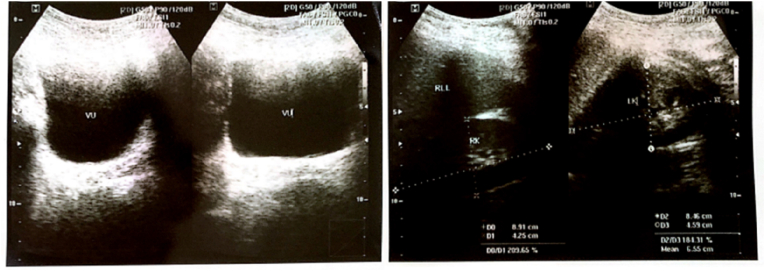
The result of Abdominal Ultrasonography.
